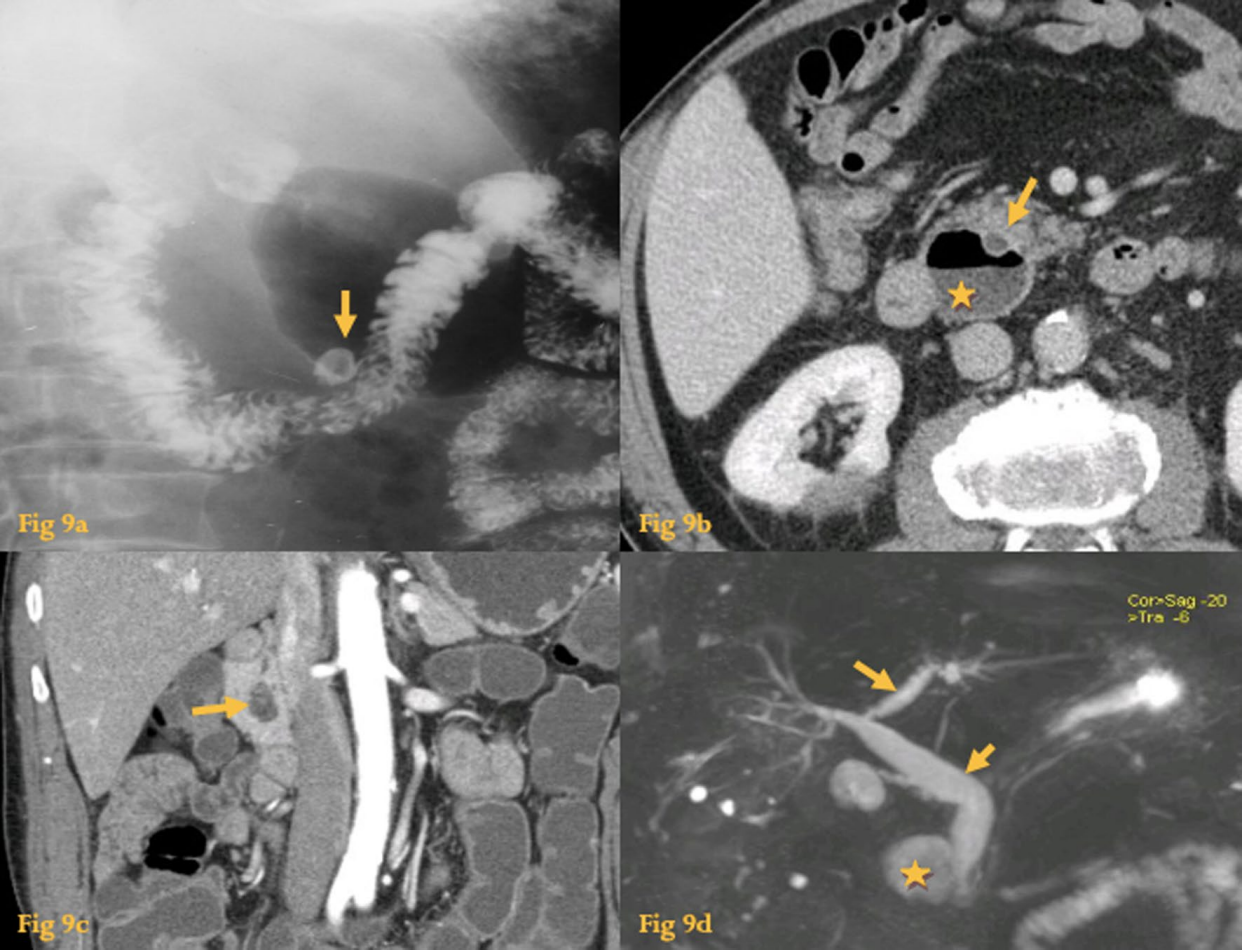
Diverticula. Upper gastrointestinal series with barium contrast (a) shows an outpouching of the upper wall of the third part of the duodenum (arrow). Abdominal CT (b) shows an air and fluid-filled diverticulum on the inner aspect of the descending duodenum (asterisk), adjacent to the CBD (arrow). In another patient (c), an heterogeneous fluid and air-filled diverticulum on the inner aspect of the descending duodenum (arrow) mimicked an abscess. MRCP (d) in a patient that had bile duct dilatation (arrows) with associated cholangitis due to a periampullary diverticulum (asterisk)
